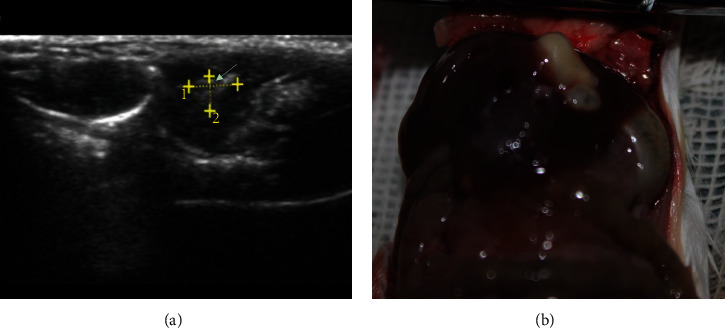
Intrahepatic AE lesion assessment. (a) Ultrasound shows a hyperechoic lesion in the liver. (b) Laparotomy shows whitish liver lesion in the left lobe.
